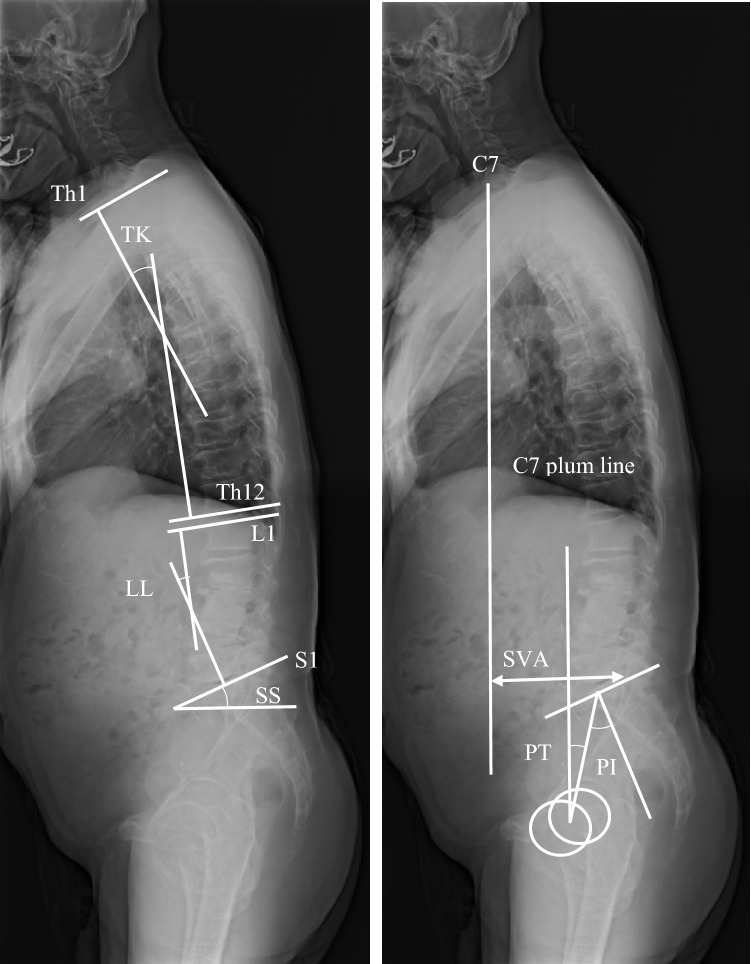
Spinal parameters on standing radiograph. Thoracic kyphosis (TK) and lumbar lordosis (LL) are defined as the angle between the cranial endplate of Th1 and the caudal endplate of Th12, the cranial endplate of L1 and the caudal endplate of L5, respectively. Sacral slope is defined as the angle between the sacral endplate and the horizontal plane. Sagittal vertical axis (SVA) is distance from C7 plumb line from the center of the C7 to the posterior edge of the upper sacral endplate. Pelvic tilt (PT) is defined as the angle is between the line connecting the midpoint of S1 endplate to the center of the femoral head and the vertical line of S1 endplate. Pelvic incidence (PI) is defined as the angle between the line perpendicular to the middle of the cranial sacral endplate and the line joining the middle of the cranial sacral endplate to the center of the femoral head axis.
